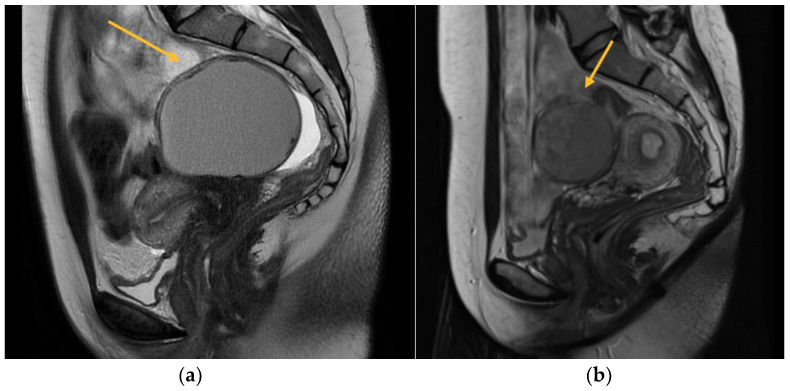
MRI aspect on T2 sagittal (a) Left endometrioma with left ovary adherent to the posterior uterine wall (b) Right endometrioma.
